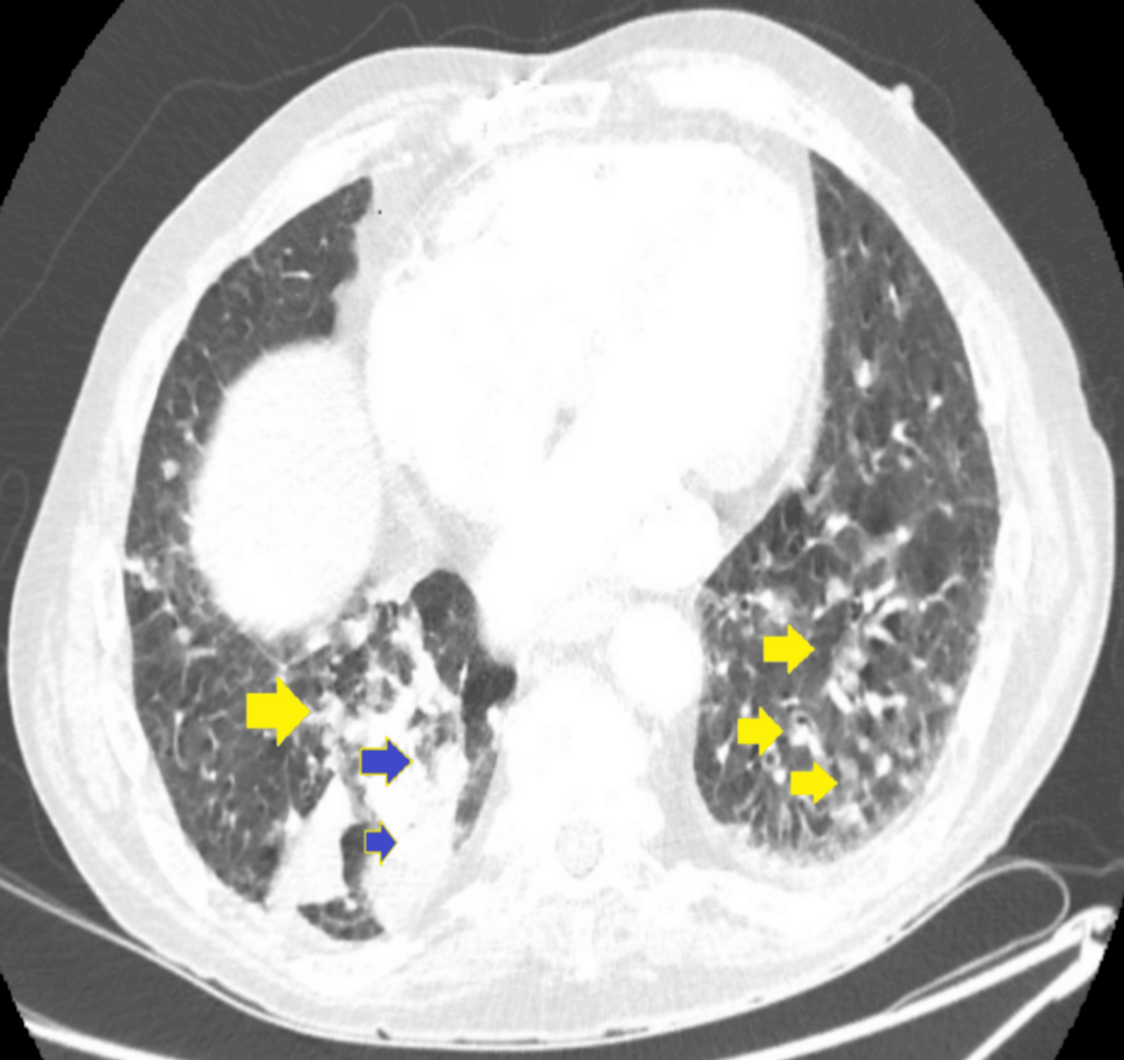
CT thorax abdo pelvis showing primary lung malignancy with extensive bilateral pulmonary metastases. 1. Yellow arrows show extensive bilateral pulmonary metastases. 2. Blue arrows show a malignant-looking mass in the right lower lobe measuring approximately 52 x 45 mm.
